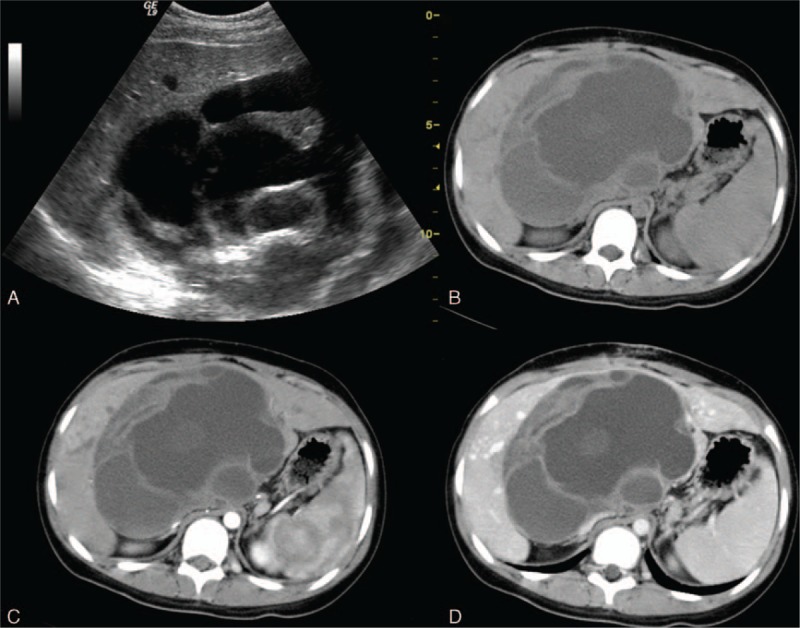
An 11-year-old girl with a mesenchymal hamartoma who presented with abdominal fullness. A, Combined cystic and solid mass is depicted on sonography. Dynamic CT with precontrast (B), arterial phase (C), portovenous phase (D) shows progressive enhancement of the septated and stromal parts of the tumor. The cystic part is without enhancement in all phases.
